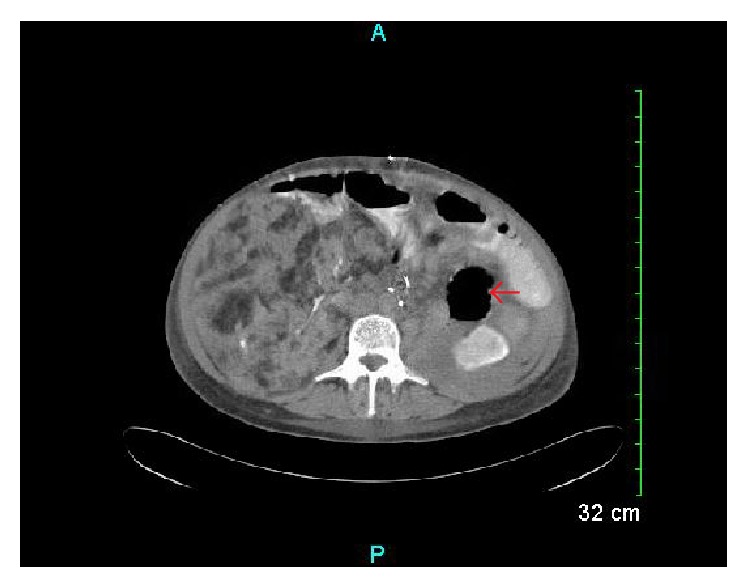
Transverse section of CT abdomen-pelvis after left nephrectomy (indicated by arrow).
